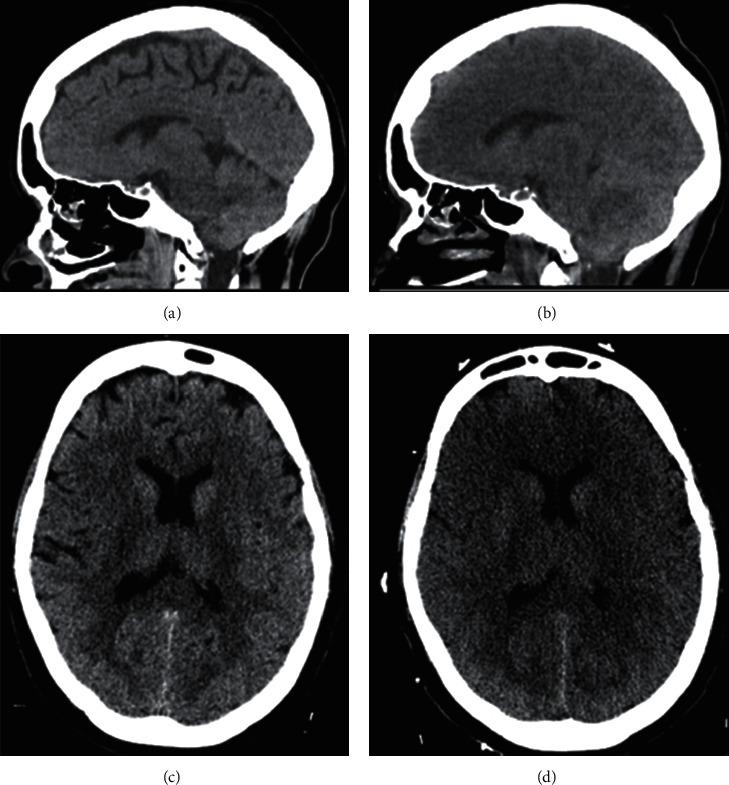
Sagittal and axial head CT performed before and 12 hours after the patient developed severe hyperammonemia. (a, b) Normal sagittal and axial brain CT. (c, d). Repeat head CT after clinical deterioration revealed diffuse hypodense cytotoxic edema with compression of the lateral ventricles and sulcal effacement. Sagittal view demonstrates interval compression of the subarachnoid cisterns and cerebellar tonsil herniation.
